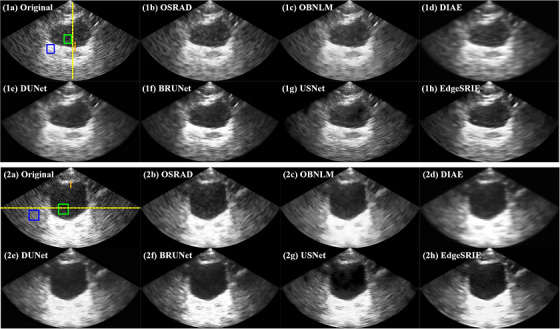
Speckle‐reduction results for two representative bladder ultrasound frames acquired with the portable EdgeFlow UH‐10 device. Rows correspond to two cases, and columns show the original image followed by OSRAD,^16^ OBNLM,^20^ DIAE,^26^ DUNet,^26^ BRUNet,^26^ USNet,^27^ and EdgeSRIE. Dashed yellow and orange lines indicate profiles used for intensity and AGM analysis, and green and blue boxes indicate ROIs used for contrast evaluation. The same display range is used within each case.
